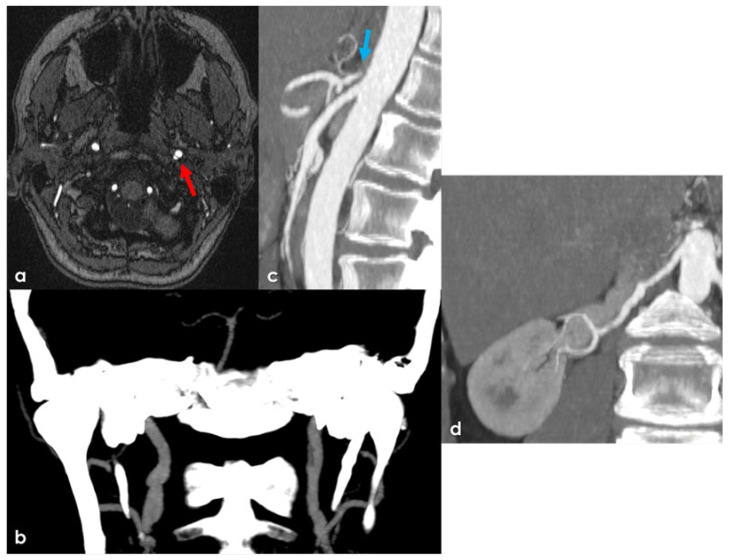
Example of a patient with LV involvement and a systemic disease (patient 7). Panel (a): brain magnetic resonance angiography (MRA) source image with a dual lumen in the left prepetrosal ICA (red arrow) as sign of dissection. Panel (b): CT angiography (CTA) processed with maximum intensity projection/multiplanar reconstruction (MIP/MPR) protocol in a coronal plane, showing the right ICA stenoses followed by vessel enlargement and the left ICA with a pseudofenestration pattern. Panel (c): aortic CTA (MIP/MPR in sagittal plane), showing a severe stenosis at the origin of the celiac trunk (blue arrow) and a fusiform aneurysm on the superior mesenteric artery. Panel (d): CTA (MIP/MPR in coronal plane) of the right renal artery, showing several irregularities in the vessel profile and a fusiform ectasia in the mid-portion.
